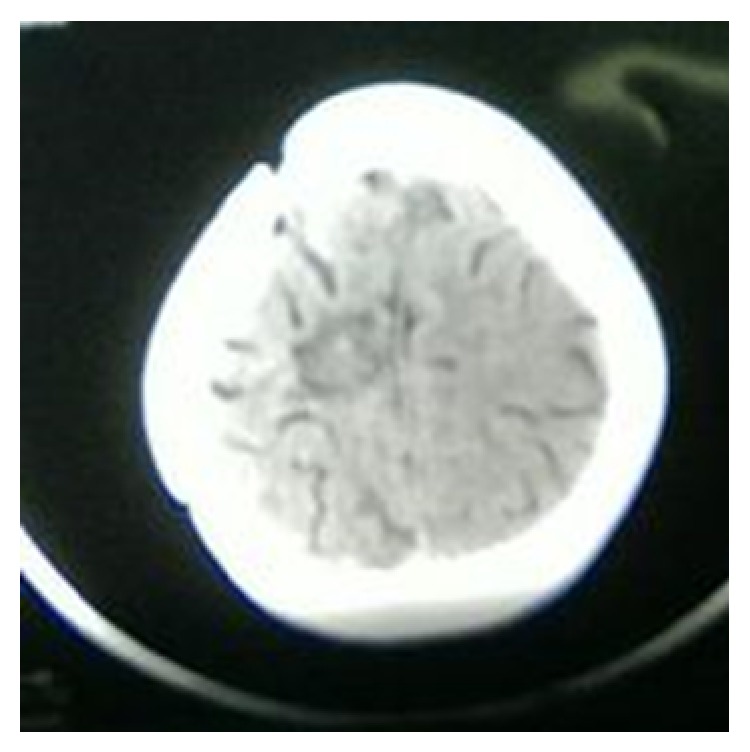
Post-op CT scan brain with residual disease.
